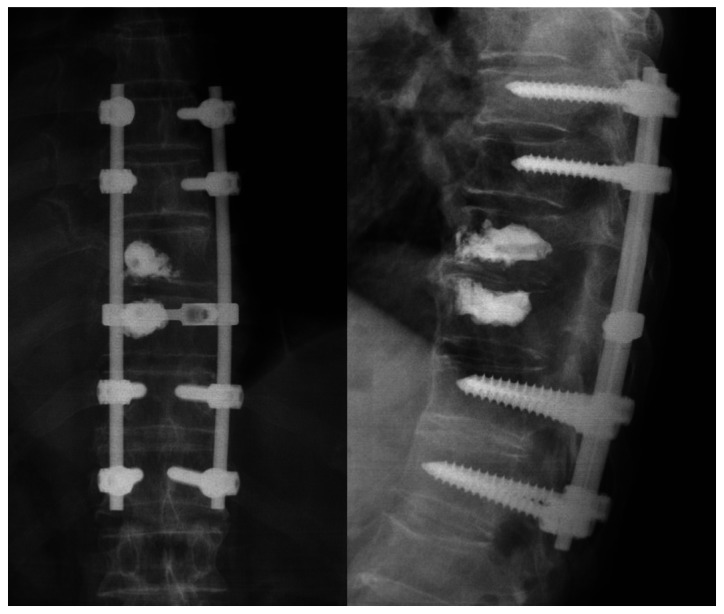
The AP and lateral X-ray postoperatively showed no cement leakage or mislocation of screws.
